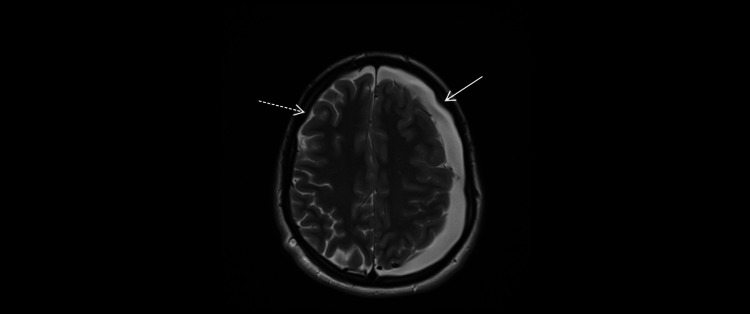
MRI (July 23, 2025; axial T2-weighted; 4-mm slices; 0.5-mm gap). Overall stability of the bilateral convexity subdural hygromas compared with the prior study: the right side remains resolved (dashed arrow), and a residual collection persists on the left without interval increase (solid arrow).
